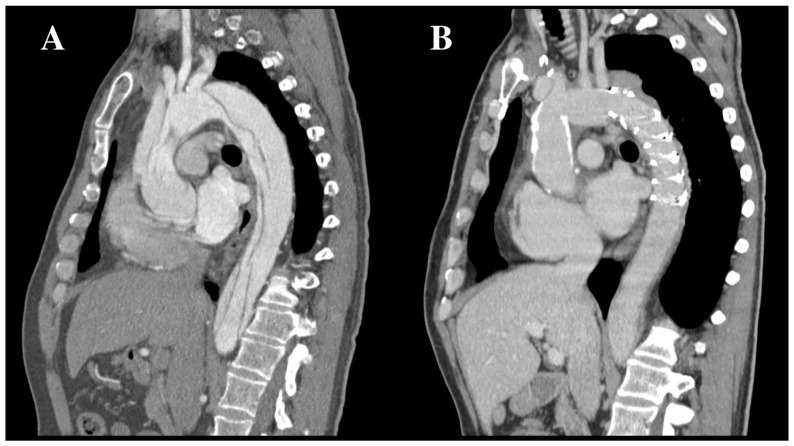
Positive aortic remodeling after frozen elephant trunk procedure. Preoperative (A) and 3-months post-operative (B) CTA of a 56-year-old male patient who experienced type A AAD and underwent emergent TAR-FET. Follow-up CTA highlighted the beneficial effect of FET on the shrinkage of the FL in the downstream aorta. AAD: acute aortic dissection; CTA: computed tomography angiography; FET: frozen elephant trunk; FL: false lumen; TAR: total arch replacement.
